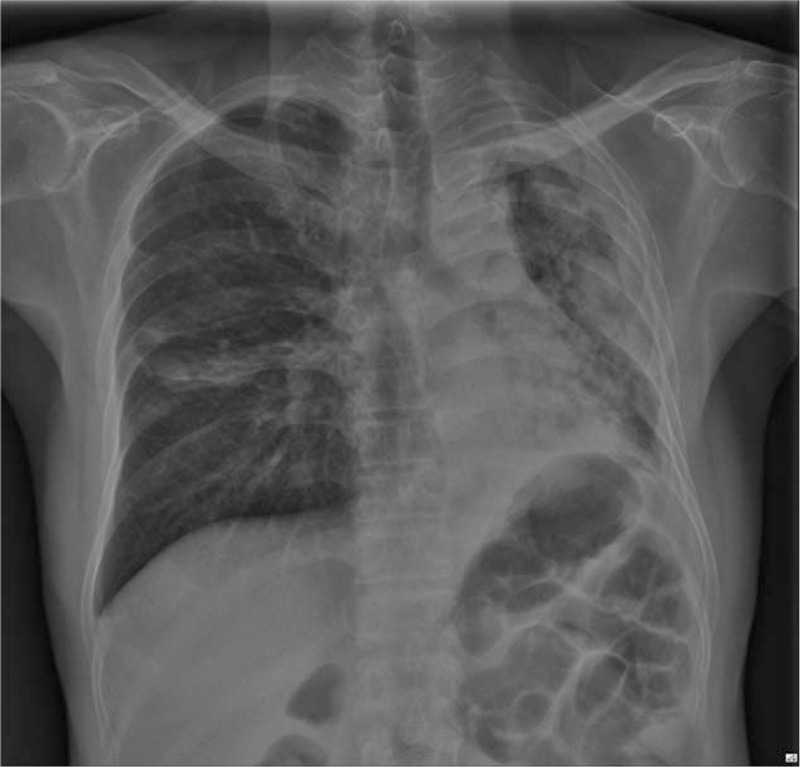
Preoperative chest x-ray (posteroanterior view).
